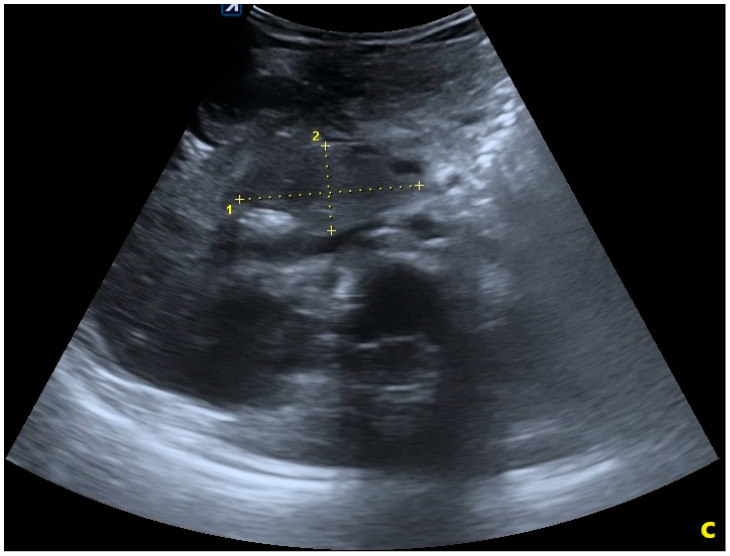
Abdominal ultrasound performed on day 2 post-injury. (a) The solid yellow arrow indicates the pancreas. The open yellow arrow identifies peripancreatic fluid in the lesser omental bursa. (b) The yellow oval delineates the progressively hypoechoic pancreatic head, reflecting evolving post-traumatic parenchymal changes. (c) Calipers delineate the inhomogeneous area within the pancreatic head, measuring 48 × 23 mm (CC × AP).
